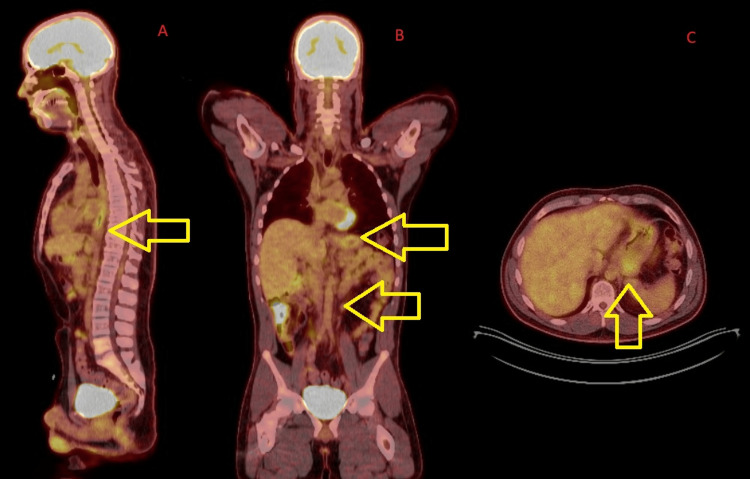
PET/CT showing complete metabolic response five months after completing concurrent chemo-immunotherapy with radiation The yellow arrows show the complete metabolic resolution of the previous sites of cancer. (A) Sagittal view. (B) Coronal view. (C) Axial view.
